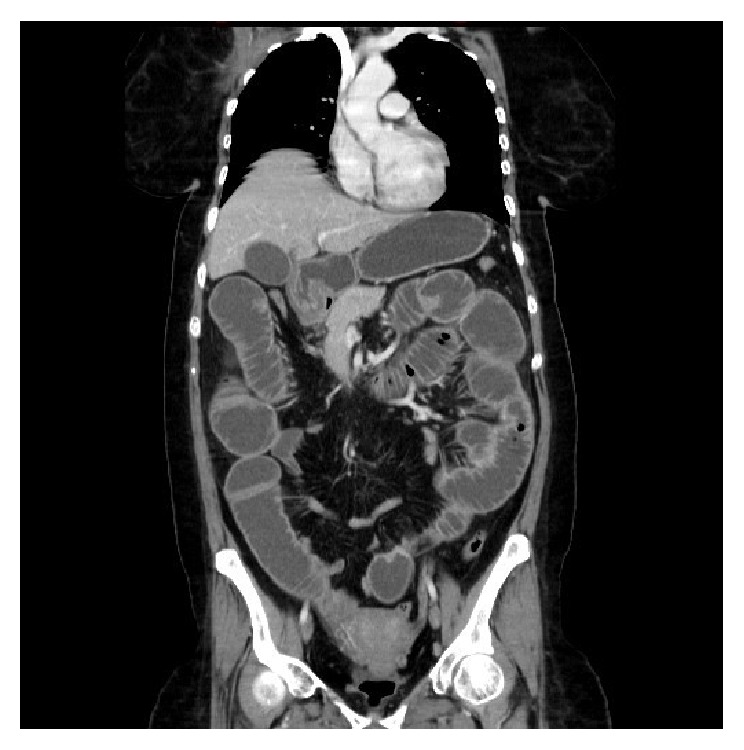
Enhanced coronal CT scan of the abdomen demonstrates mild circumferential wall thickening with luminal narrowing involving the second part of the duodenum associated with surrounding fat stranding.
